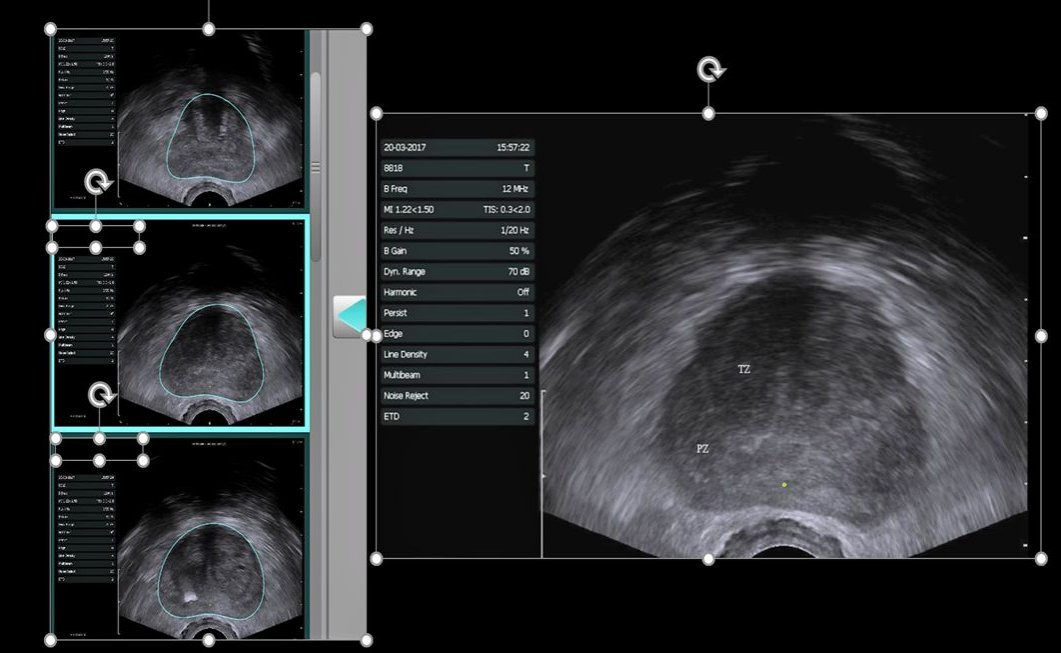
Transrectal Ultrasound Normal TRUS in axial plane shows transitional zone (TZ) and peripheral zone (PZ) without focal hypoechoic nodules. Scanning is volumetric and can be depicted in all three planes: axial, sagittal, and coronal.
